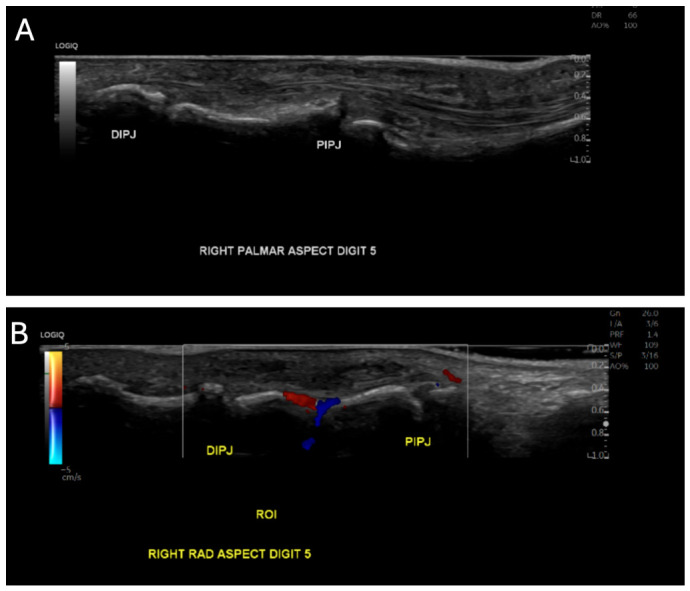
Initial ultrasound imaging of the right little finger. (A) Palmar aspect ultrasound demonstrating the lesion within the right little finger. (B) Radial aspect ultrasound of the right little finger demonstrating the lesion from a radial approach.
